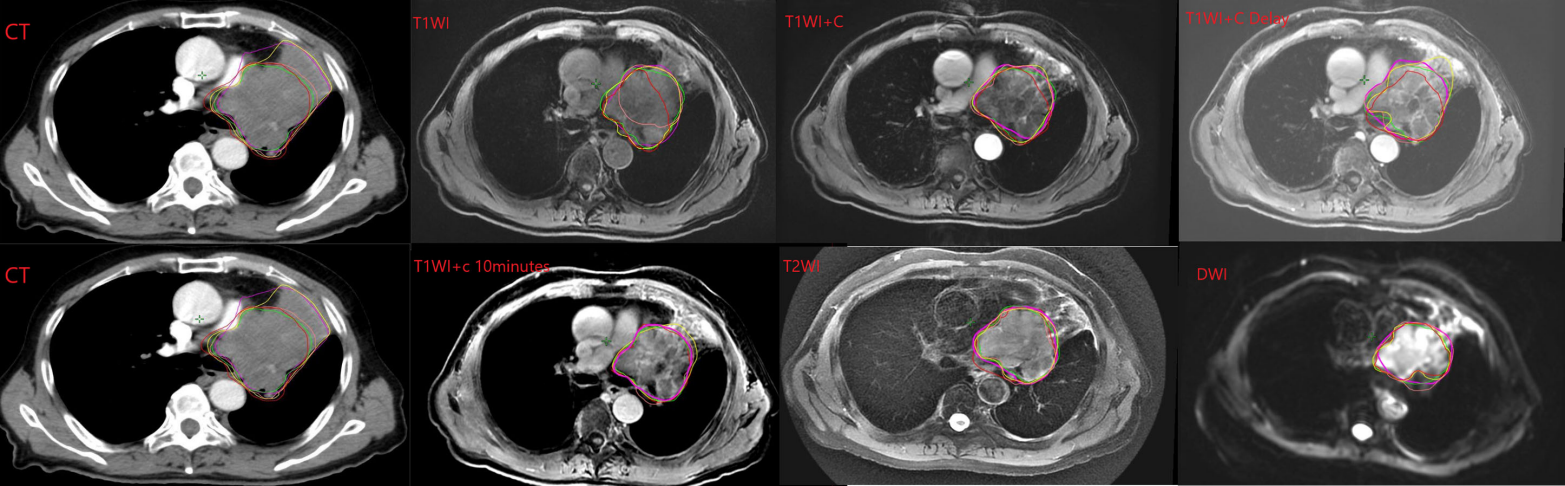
Example of GTV contoured on the different CT and MRI sequences by each observer. Note: The lines of different colors in the picture represent different observers.
